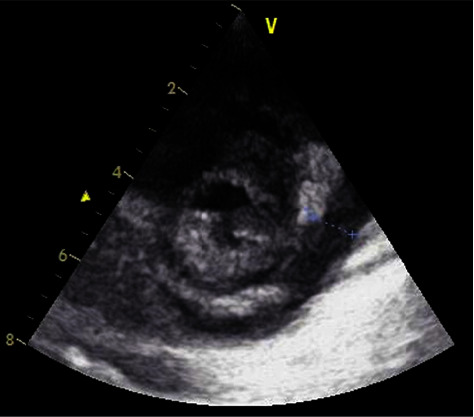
Parasternal short-axis view of transthoracic echocardiograms during the acute illness revealing 8.6-mm pericardial effusion thickness.
